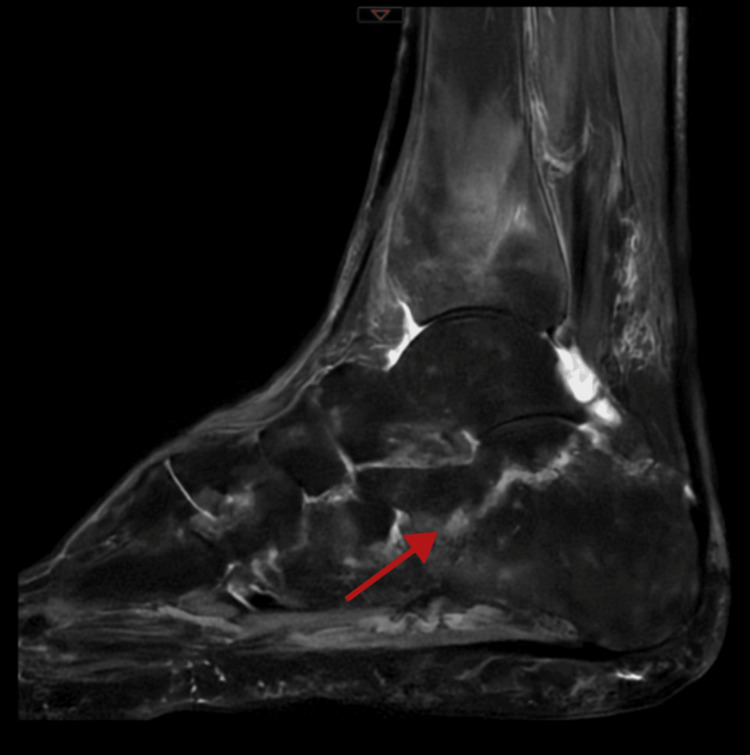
T2-weighted MRI of the right calcaneus showing bone marrow edema with features suggestive of a stress fracture (red arrow) MRI: magnetic resonance imaging
